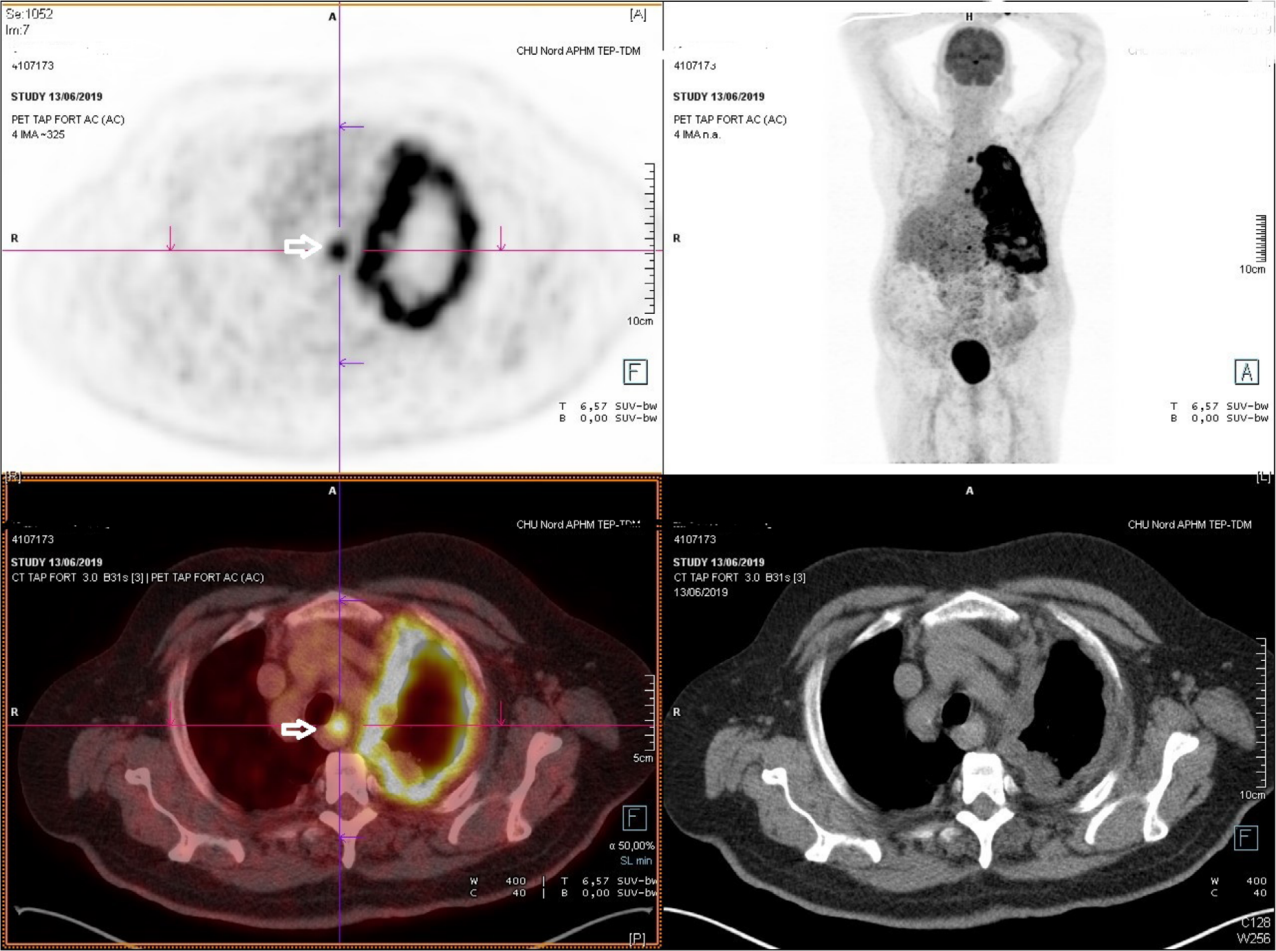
Evaluation of the patient by 18F‐fluoro‐2‐deoxy‐d‐glucose positron emission tomography/computed tomography fused imaging mimicking a malignant pleural mesothelioma with a circumferential hypermetabolic thickening of the pleura in the left pleural space. A hypermetabolic homolateral mediastinal lymph node is also visible (arrow).
